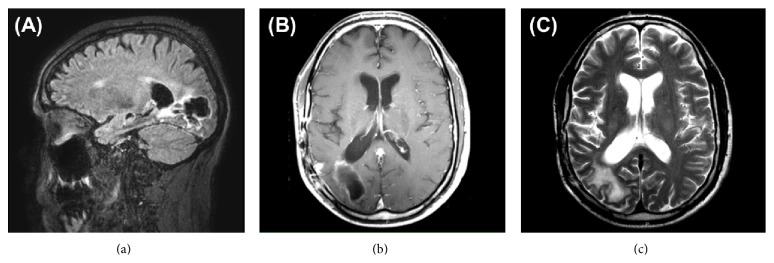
Intracranial imaging during final course of salvage therapy with bevacizumab and carboplatin. (a) FLAIR, (b) T1-weighted axial, and (c) T2-weighted axial magnetic resonance imaging demonstrating stable intracranial disease at time of respiratory decline. Postsurgical hyperintensity surrounds area of resection in the right temporal and occipital lobes communicating with the right occipital horn, unchanged with respect to prior imaging.
